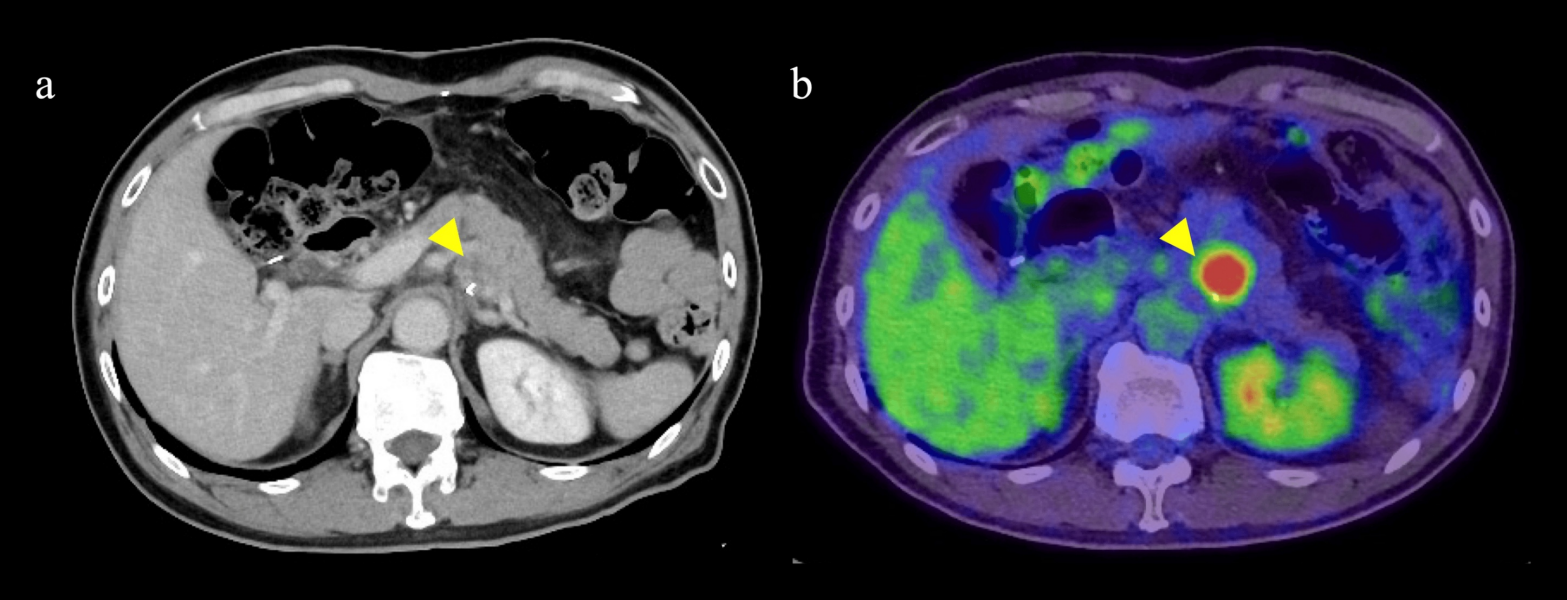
Images of recurrent lymph nodes. (a) Enlarged lymph nodes on the ventral side of the superior mesenteric artery (yellow arrowhead). (b) PET-CT shows abnormal fluorodeoxyglucose (FDG) accumulation in the same region (yellow arrowhead).
